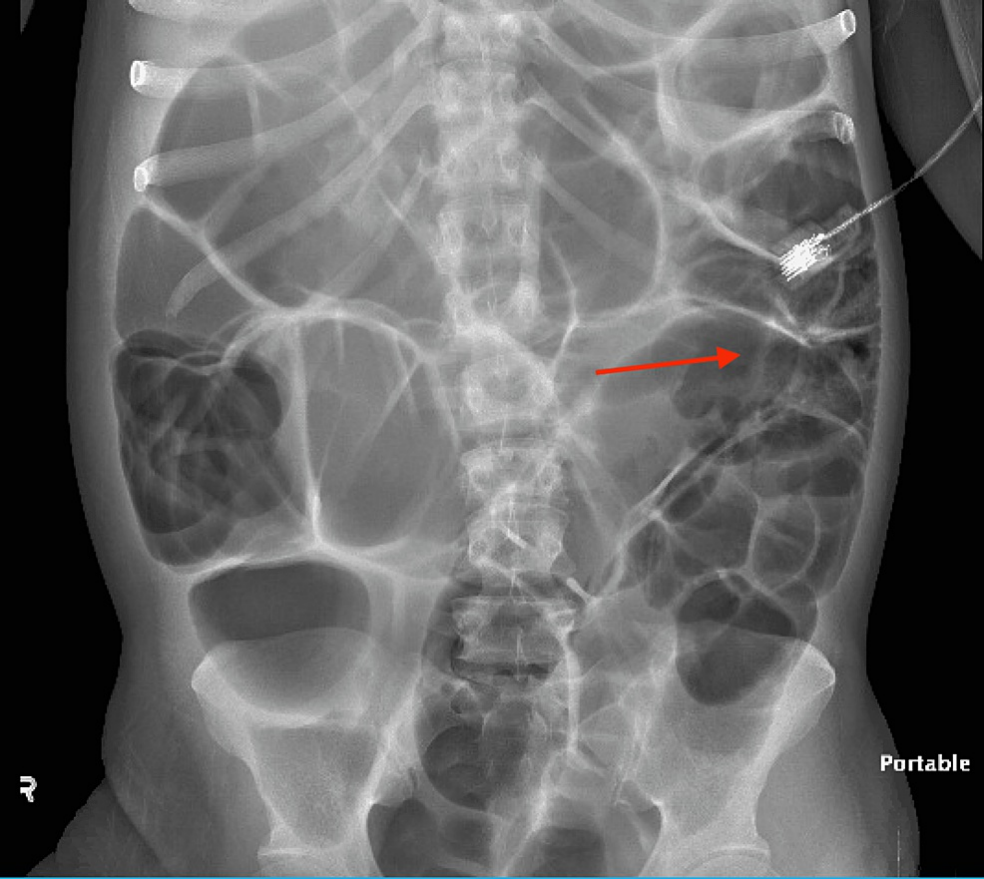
Abdominal X-ray with red arrow showing diffuse large and small bowel gas most suggestive of ileus
